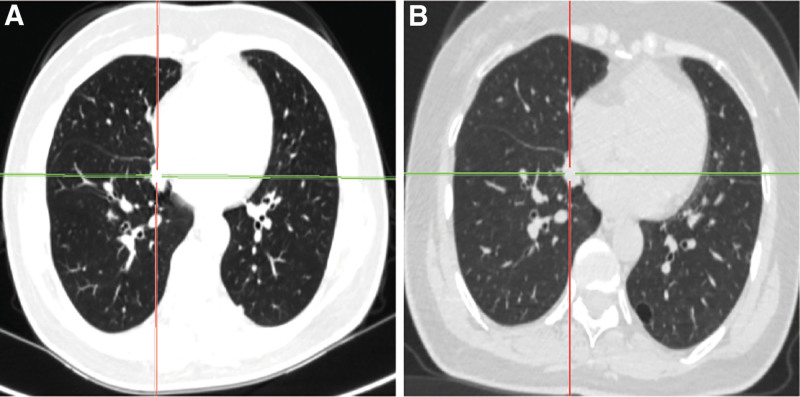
(A) January 2024 chest CT lung window; (B) April 2024 chest CT lung window. CT = computed tomography.
